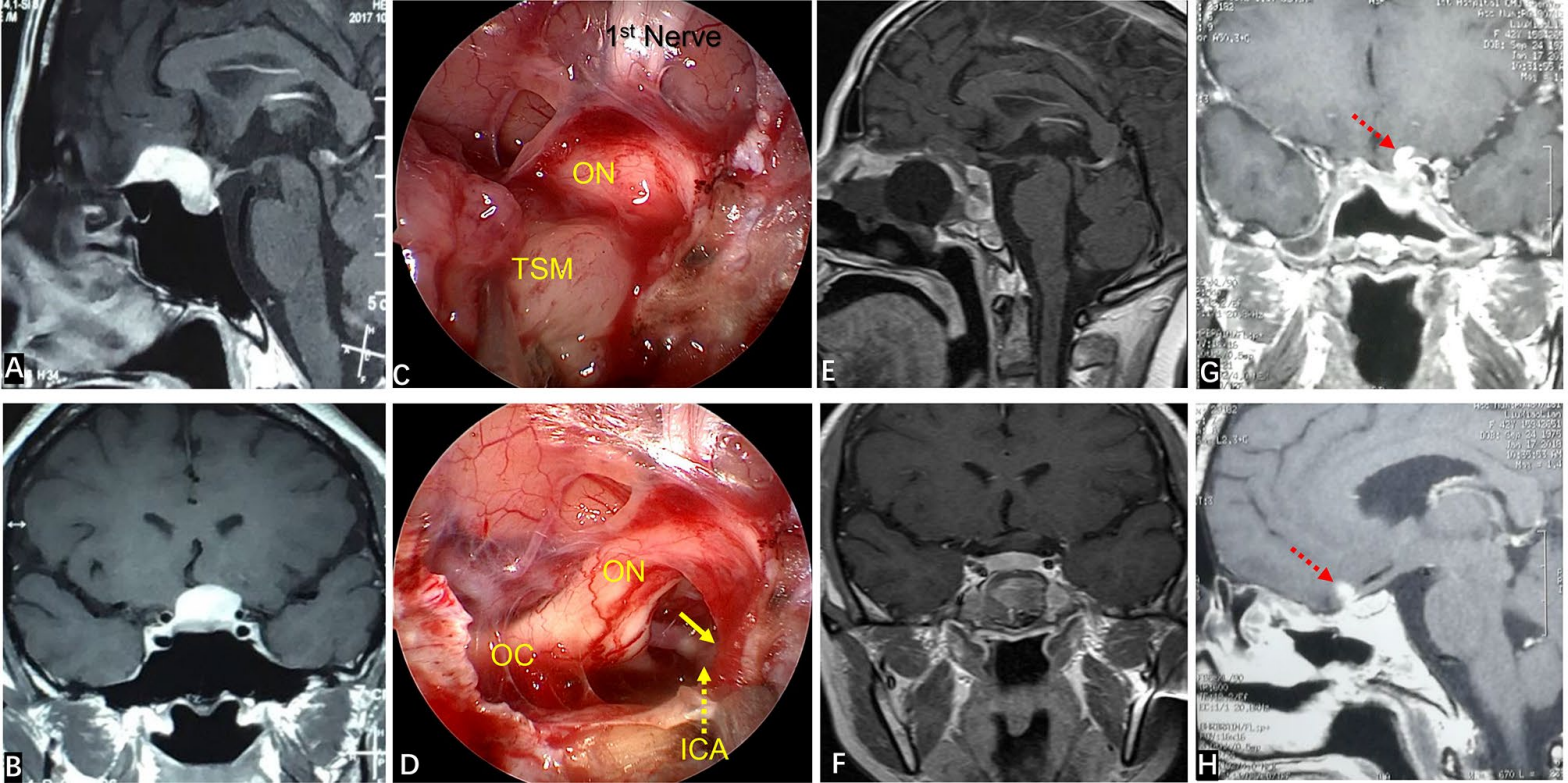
(A,B) Preoperative contrast-enhanced MRI of the sellar region showed the tumor extending along the sphenoid platform. (C,D) Intraoperatively, the tumor adhered closely to the left optic nerve and ICA. After tumor removal, little residual tumor remained at the left ICA (yellow solid-line arrow). (E,F) At POD5, contrast-enhanced MRI of the sellar region showed that the tumor has been resected completely and that no residual tumor remained at the left ICA. (G,H) Three months after surgery, contrast-enhanced MRI showed that a small tumor mass remained in the left ICA (red dotted-line arrow).
